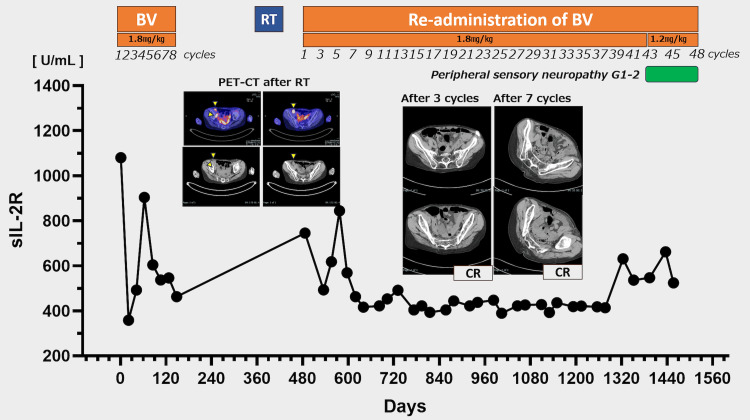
Clinical course of brentuximab vedotin administration. During both the initial administration and re-administration of BV, serial measurements of soluble interleukin-2 receptor (sIL-2R) demonstrated a progressive decline. Following re-administration, CT evaluation revealed CR after three cycles. Regular surveillance CT examinations performed at three-month intervals confirmed sustained remission status throughout the extended treatment period.
